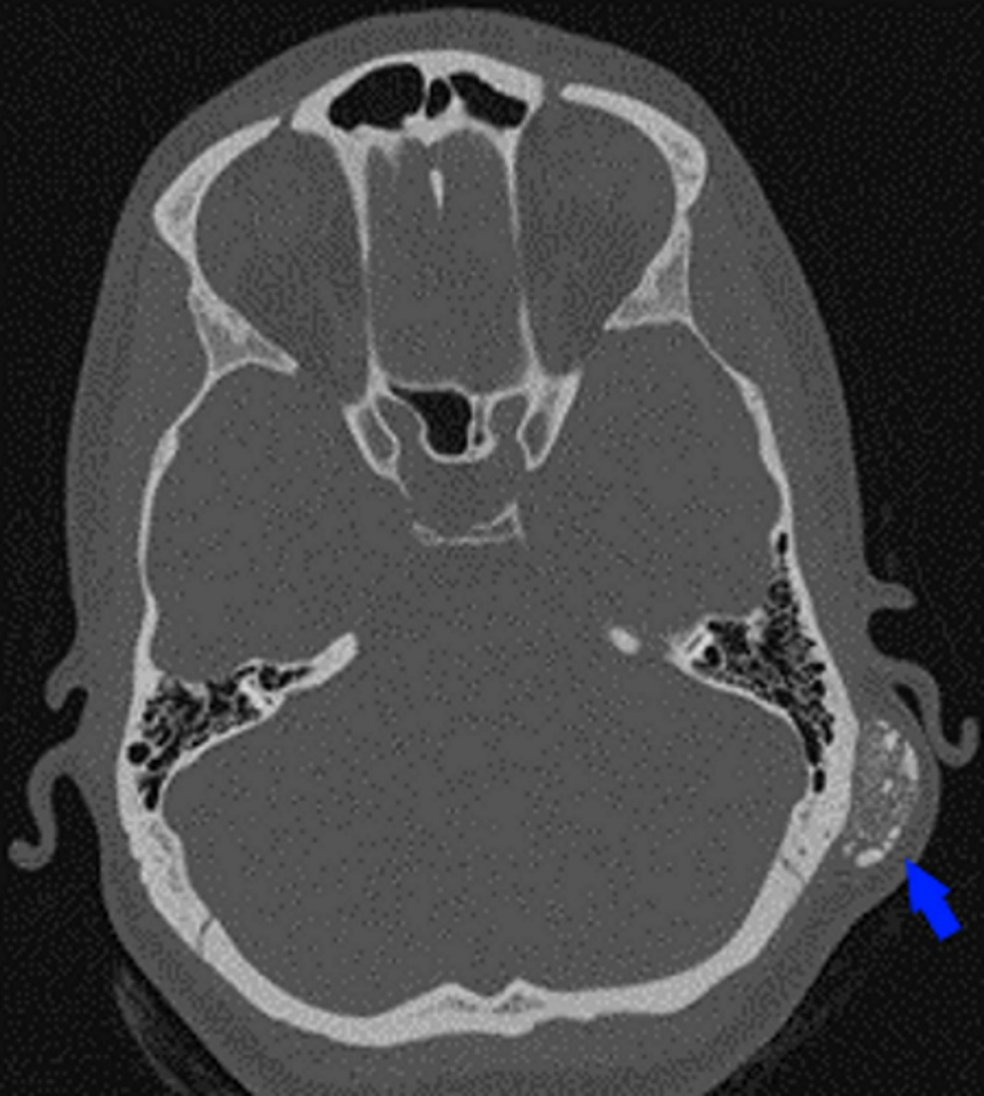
Temporal bone CT image of the lesion (blue arrow), axial section. CT: computed tomography.
